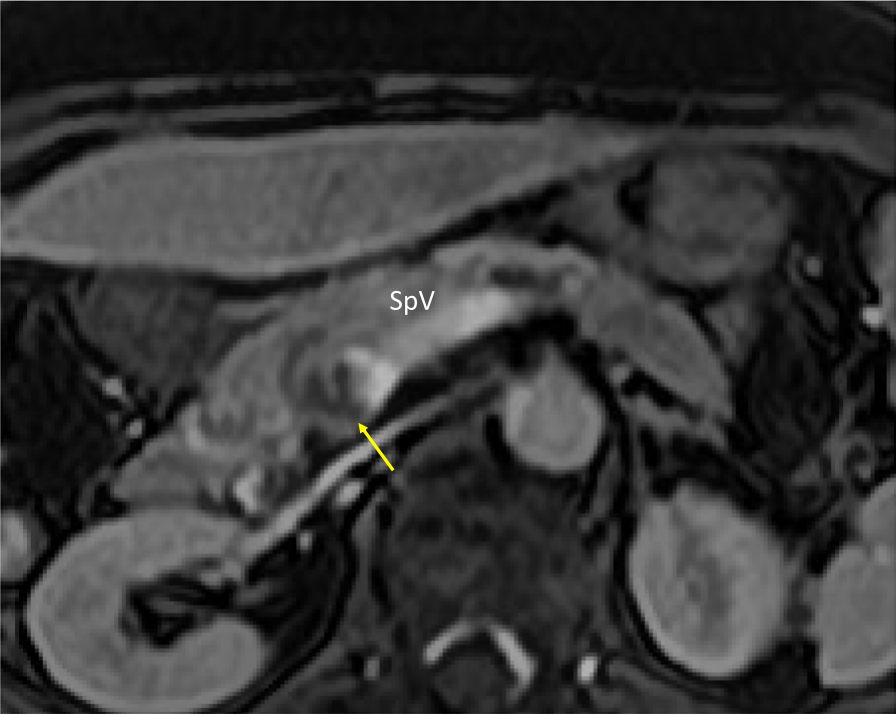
Magnetic resonance imaging 1 week after contrast-enhanced computed tomography showing the tumor emboli extending to the confluence of the superior mesenteric vein/splenic vein
